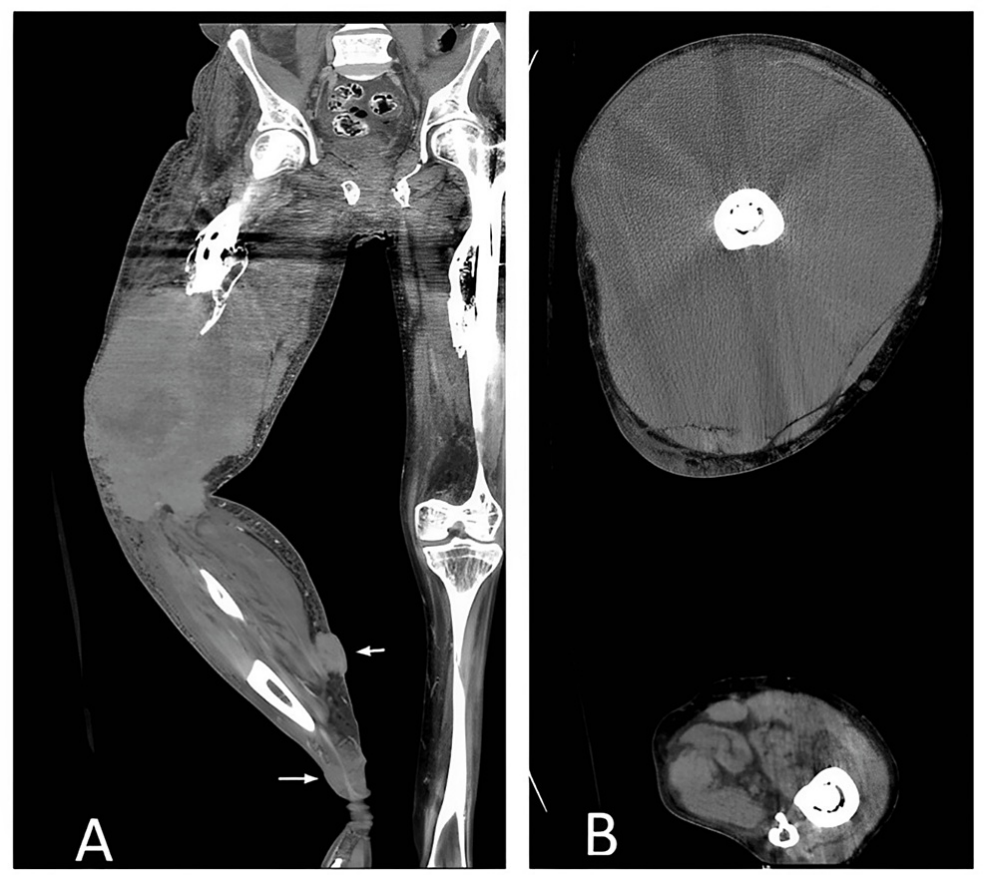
Computed tomography scan of the thigh showing a large heterogeneous soft-tissue mass measuring approximately 19.8 × 18.5 × 35 cm.
